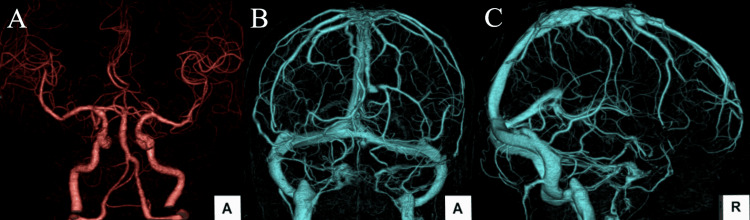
Three-dimensional CT angiography (CTA) and CT venography (CTV) demonstrating no vascular abnormalities. (A) Three-dimensional CTA shows no evidence of intracranial aneurysm, vascular malformation, or arterial dissection. (B) Frontal view of three-dimensional CTV demonstrates normal cerebral venous structures without evidence of venous sinus thrombosis. (C) Lateral view of three-dimensional CTV also shows no evidence of venous sinus thrombosis.
